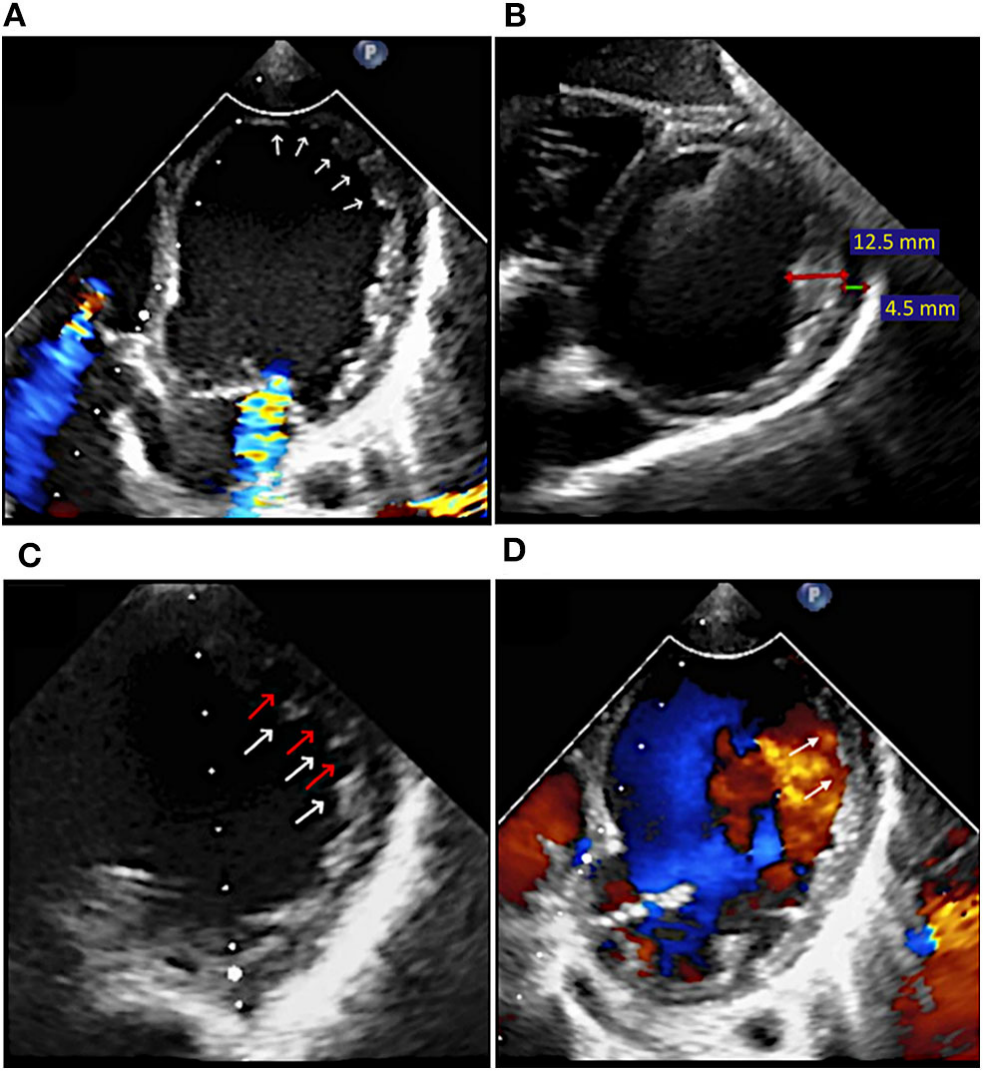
Case 1: Transthoracic echocardiography at the moment of diagnosis: (A) Apical and lateral LV trabeculations and intertrabecular recesses (arrows); (B) Non-compaction (red line) to compaction (green line) ratio in end-systole of >2.7 (12.5/4.5 mm); (C) LV trabeculations (white arrows) and intertrabecular recesses (red arrows) more visible in diastole; (D) Color Doppler echocardiography showing direct blood flow from LV cavity into the recesses (arrows) in diastole.
